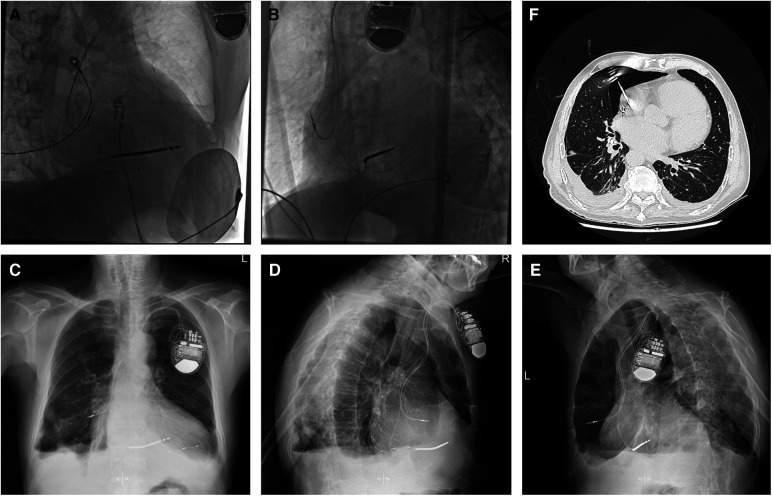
Fluoroscopy images of the initial active fixation lead in RA free wall of case 1 [(A) RAO view and (B) LAO view]. Imaging examinations of case 1 obtained on readmission (C–F). Chest radiograph indicating the atrial lead protruding outside the contours of RA [(C) AP view, (D) RL view, and (E) LL view]. Chest CT scan showing the tip of the atrial electrode touching the right upper lobe of the lung and causing pneumothorax (F). AP, anterior posterior; CT, computed tomography; LAO, left anterior oblique; LL, left lateral; RA, right atrium; RAO, right anterior oblique; RL, right lateral.
